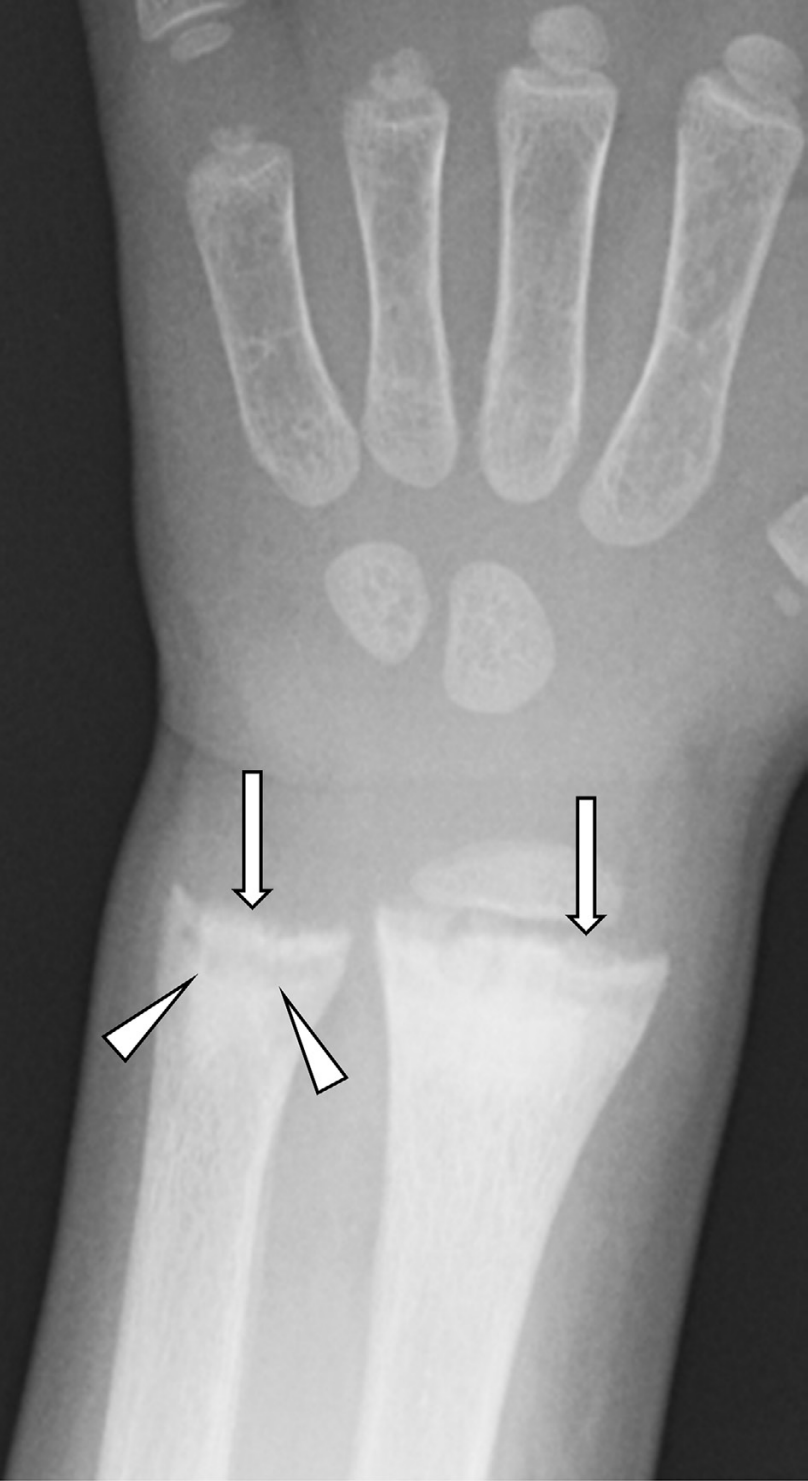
Rickets in the wrist. A 3-year-old boy who was an extremely picky eater and had a cognitive delay. He always stayed at home and rarely played outside. Anteroposterior radiograph of the left wrist showed concave deformity of the growth plate of the ulna (cupping: arrowheads) and an indistinct metaphyseal margin (fraying: arrows) in the radius and ulna. His activated vitamin D level (<5 pg ml^−1^) was lower than the paediatric normal range (20–70 pg ml^−1^).
